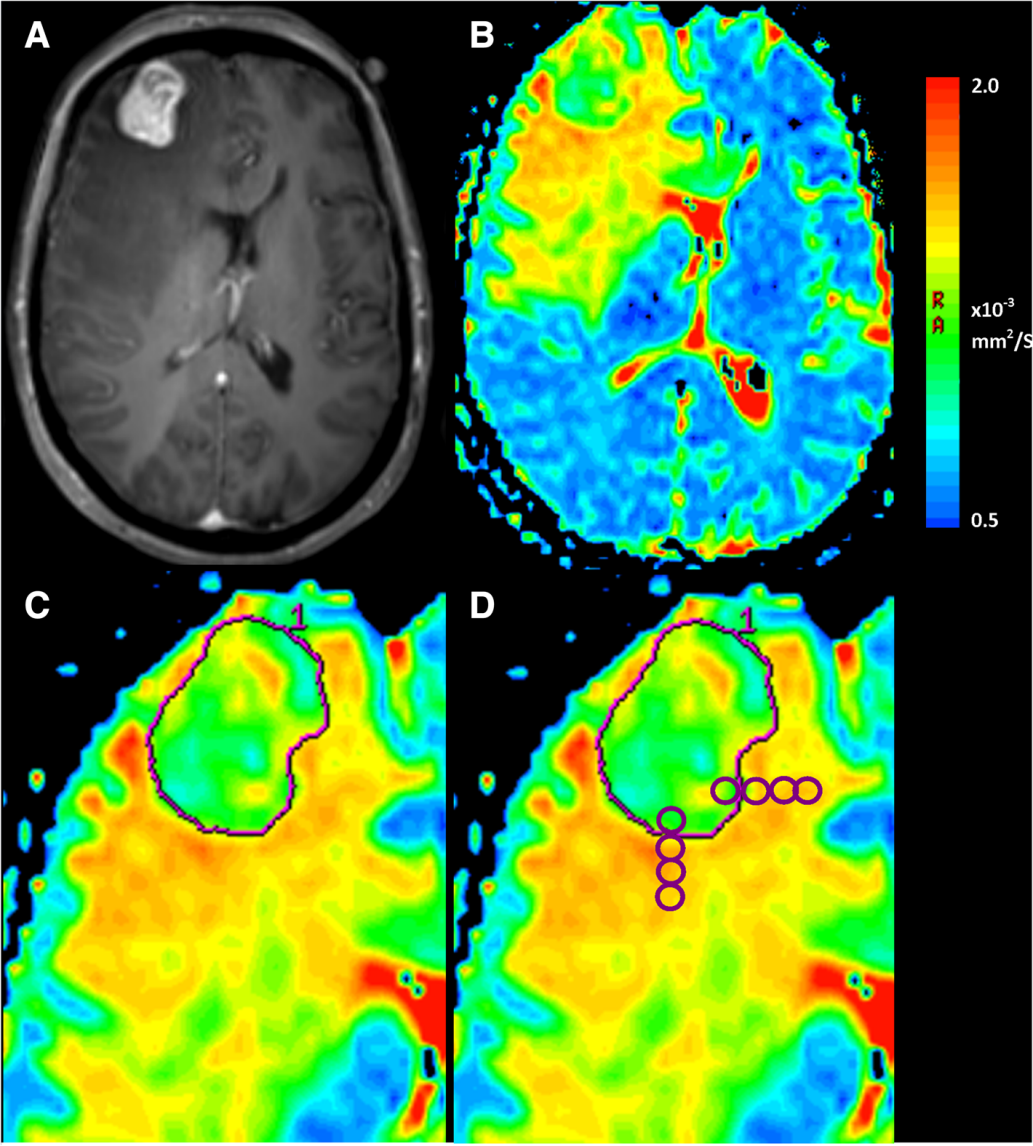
Measurement of ADC metrics from brain metastases on diffusion-weighted MRI scans. A patient with a history of lung adenocarcinoma presents with headache and focal neurological deficit. A. Postcontrast T1-weighted sequence demonstrates a right frontal lesion, which was confirmed as a metastasis after excision. B. An ADC map is generated from B = 0.1000 images using post processing software. On this color map, blue areas represent low ADC and red higher ADC. C. A freehand region of interest is traced around the tumor border using the postcontrast T1-weighted sequence as reference on the axial slice with the largest tumor area and those immediately above and below. The ADCmin is the lowest bin of a histogram of ADC values for all pixels contained within this ROI, averaged over these three slices. Three ROI are placed within the tumor on the axial slice with the largest area, avoiding necrosis, haemorrhage, cyst and the mean taken to give ADCmean. D. To assess change of ADC across the tumor border, four ROI are placed starting just inside the tumor border and extending out into the peritumoral region. The slope of the four ADC values is taken as the “ADC transition coefficient” or ATC. This is repeated in 3 orthogonal directions, avoiding structures such as ventricle or falx and then repeated on two slices above and below, with the ATC being the mean of the 9 readings.
